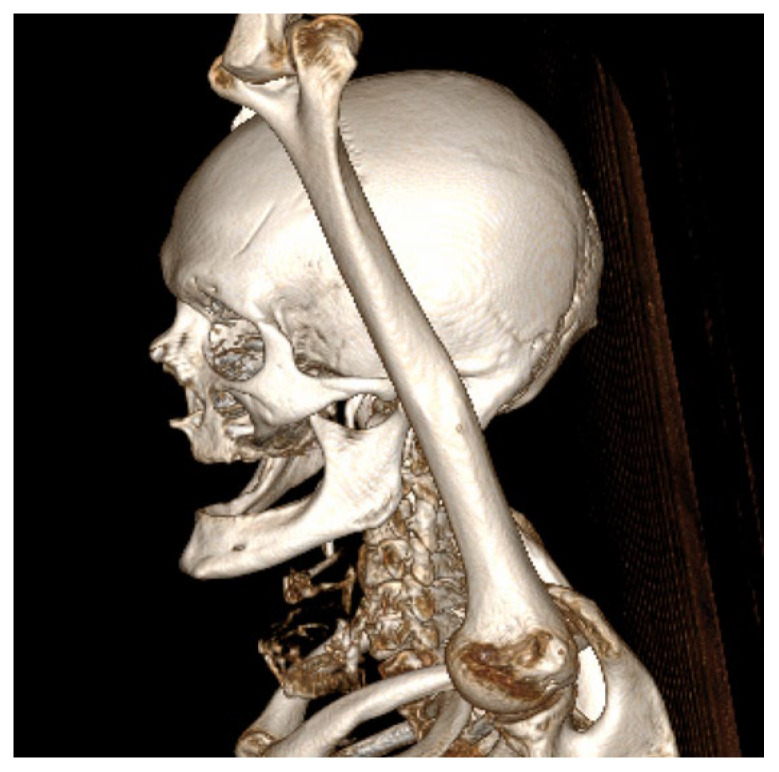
Three-dimensional CT reconstruction in left lateral view demonstrating the craniofacial skeleton, cervical vertebrae, and upper thoracic cage with no bone destruction.
